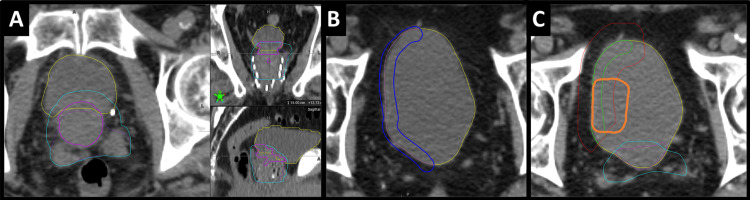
Depiction of critical structure derivations in Case 2 The above image shows how the volumes of the final targets and avoidance regions were generated. (A) Relatively inferior axial slice with the bladder (yellow), true urethra/trigone (magenta), and the resultant opti_Avoid (cyan) generated on the initial planning CT. Coronal and sagittal views are displayed as well, with the high-intensity/high-Z prostate seeds visible from previous LDR brachytherapy. (B) Axial slice showing the bladder (yellow), Brush_RtBladderWall (blue) that was manually drawn to about 10 mm thick on the right side of the bladder, and the resulting tempCTV for the right bladder wall to be treated. (C) shows the final targets: opti_CTV_RtBladderWall (green) and opti_PTV_RtBladderWall (red) that have been derived from tempCTV and cropped away from the opti_Avoid (cyan). The approximate original tumor location (delineated via registration of pre-op MRI) is shown (orange) to demonstrate the previously involved bladder wall region is encompassed by the derived targets.
